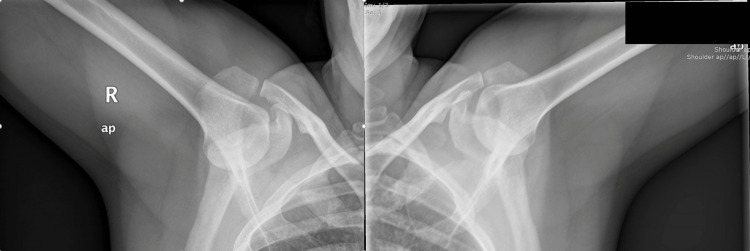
Anteroposterior X-ray showing a bilateral inferior glenohumeral dislocation
